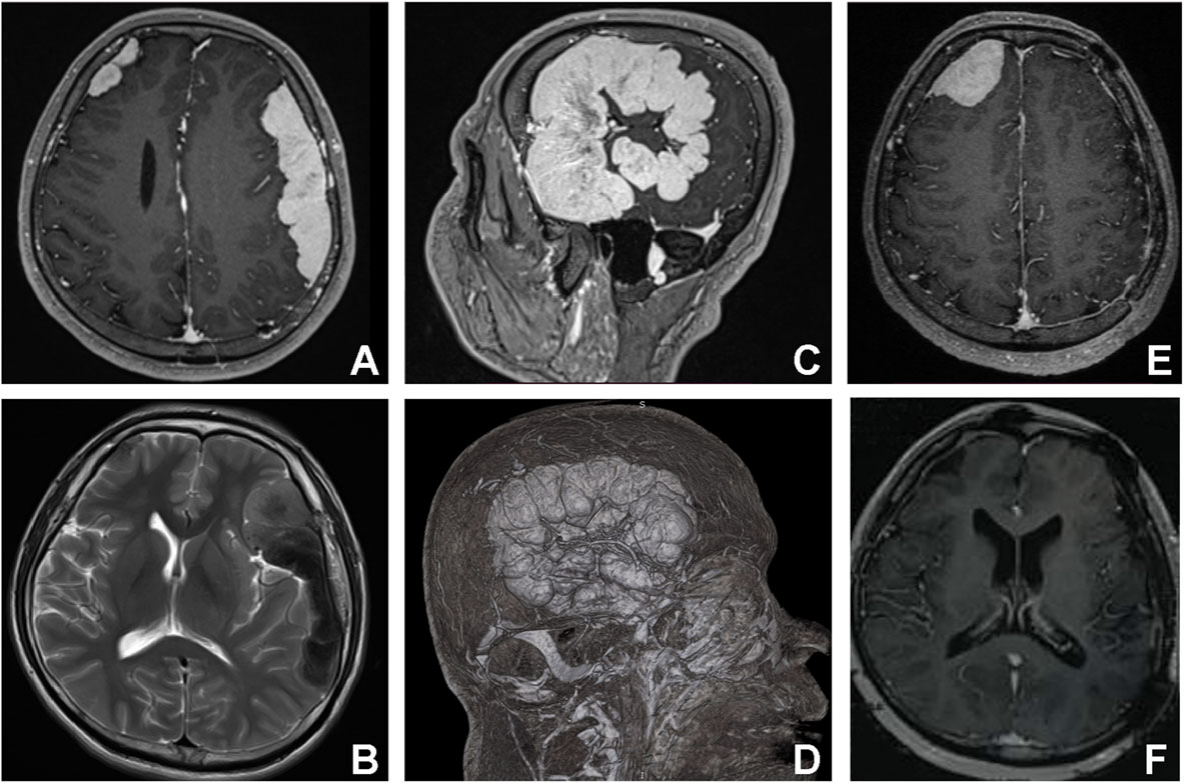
Radiological findings. A. Axial T1-weighted MRI before the first surgery demonstrates two extra-axial, homogeneous, well-defined, dural-based obviously uniform enhancement lesions, including a large lesion (93mm × 80mm × 38mm) in the left frontotemporal parietal region, and a small lesion (38mm × 35mm × 11mm) in the right frontal region. B. Axial T2-weighted MRI before the first surgery. C. Sagittal T1-weighted MRI before the first surgery. D. 3D reconstruction from images before the first surgery shows the large lesion is rich blood supply which is mainly supplied by the middle meningeal artery. E. Axial T1-weighted MRI shows the right frontal lobe lesion grows obviously (50 mm × 50mm × 25 mm) after 20 months. F. Axial T1-weighted MRI shows that there is no tumor recurred in 12 months after the second surgery
